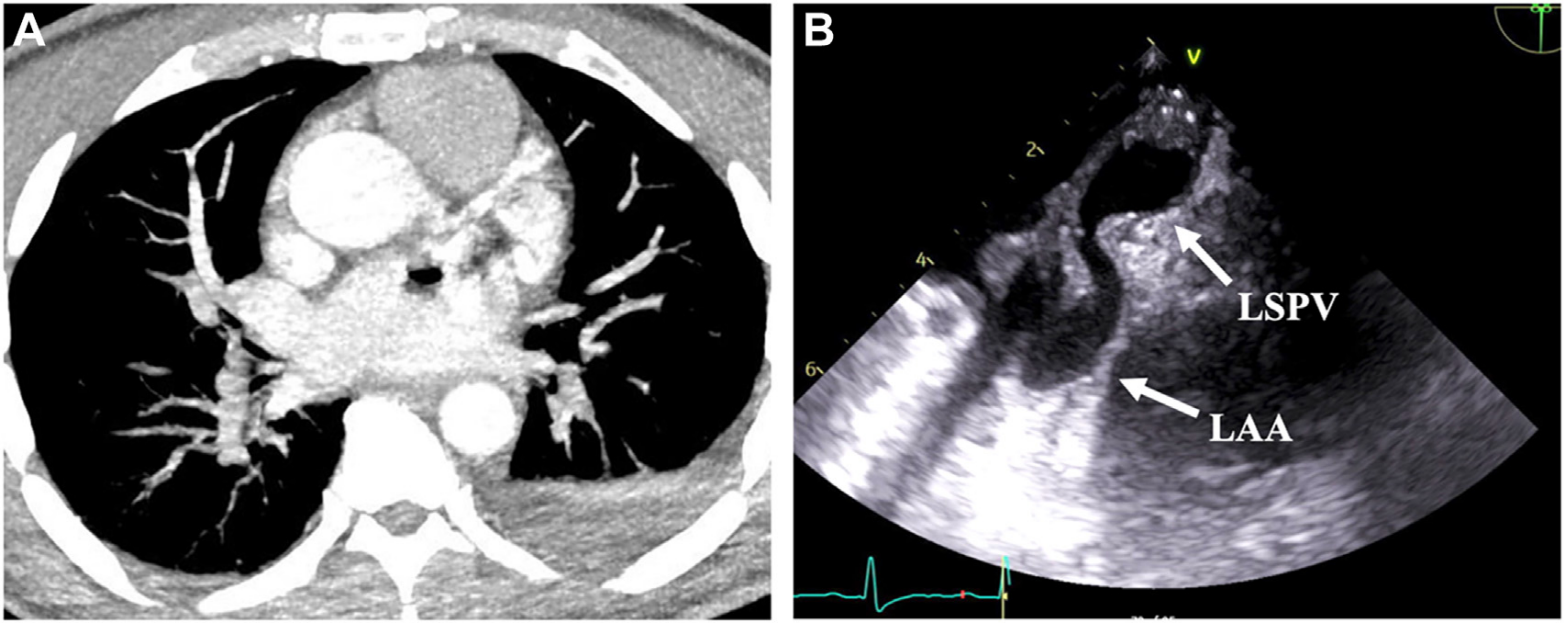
(A) Cardiac computed tomography angiograph demonstrating intracardiac air with complex left atrial mass. (B) Transesophageal echocardiogram demonstrating the atrial mass arising from the posterior atrial wall adjacent to the left superior pulmonary vein (LSPV) extending into the left atrial appendage (LAA).
